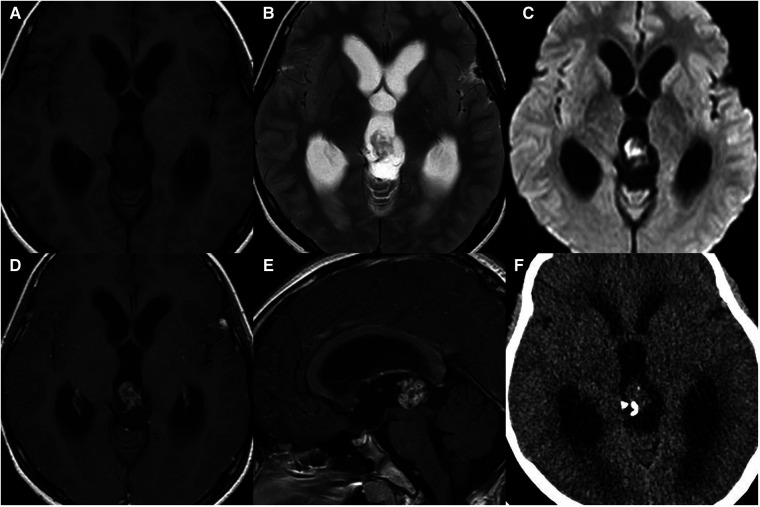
11-year-old boy with a pineal germinoma: axial T1WI (A), T2WI (B), and DWI (C), axial and sagittal post-contrast T1WI (D,E), and axial unenhanced CT (F) show a pineal mass with cystic change and engulfed calcification.
